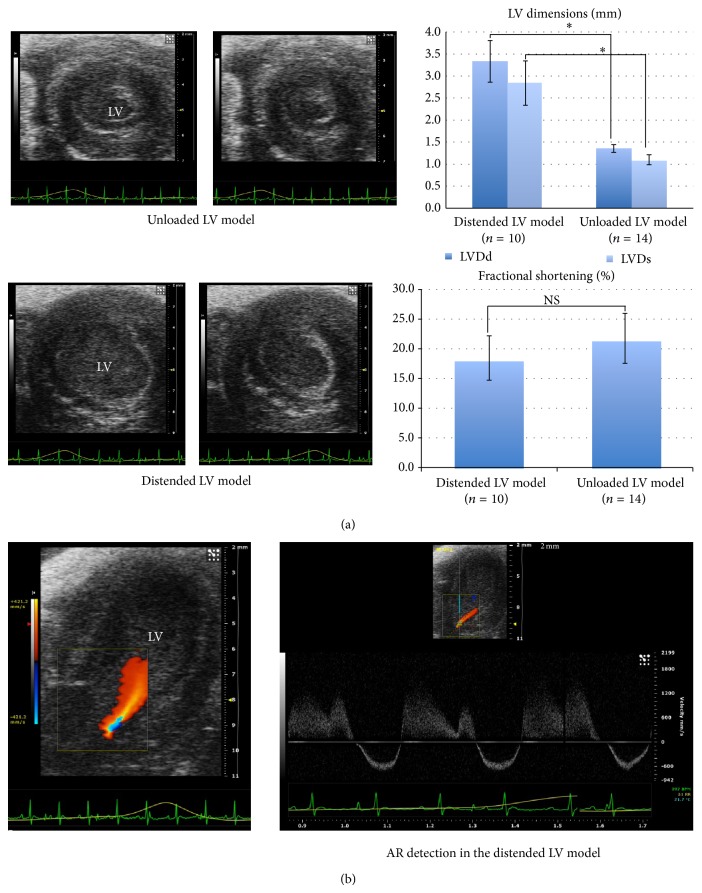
Postoperative echocardiography. (a) Short axis views of LVs in diastole and systole. The distended LV model developed significantly larger LVs than those in the unloaded LV model (^*^
